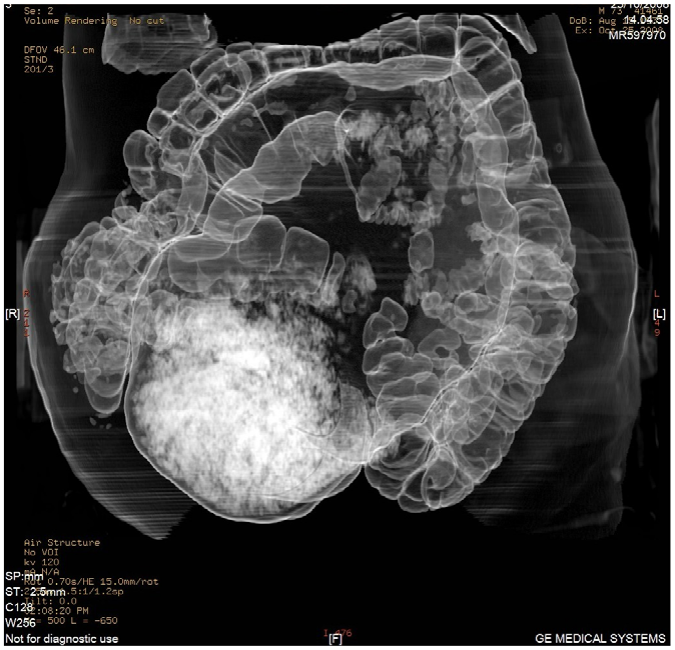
Multiplanar and 3-D reconstruction CT scan showed abnormally dilated descending colon and tortuous twisting loop, worsening the neurogenic chronic faecal stagnation.
